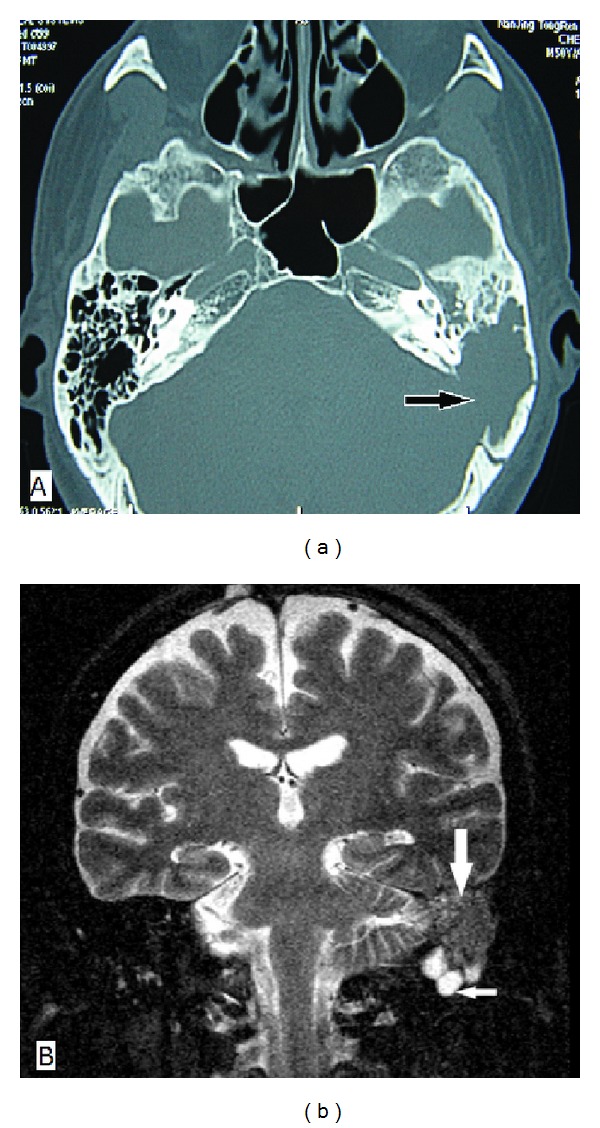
Imaging findings of Case 1. (a) Axial CT of the temporal bone shows a soft-tissue mass in the left middle ear and mastoid cavity with extension into the sigmoid sinus plate (arrow); (b) coronal MRI shows a slightly low-signal-intensity mass on T2-weighted images in the left mastoid (large arrow). Several inferior lymph nodes are seen (small arrow).
